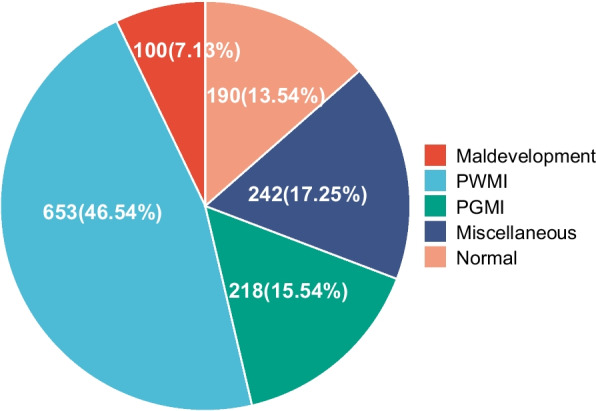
Distribution of MRI patterns. PWMI was the most common MRI pattern, observed in nearly half of the children with CP (46.54%, 653/1403), and followed by miscellaneous pattern and PGMI pattern
